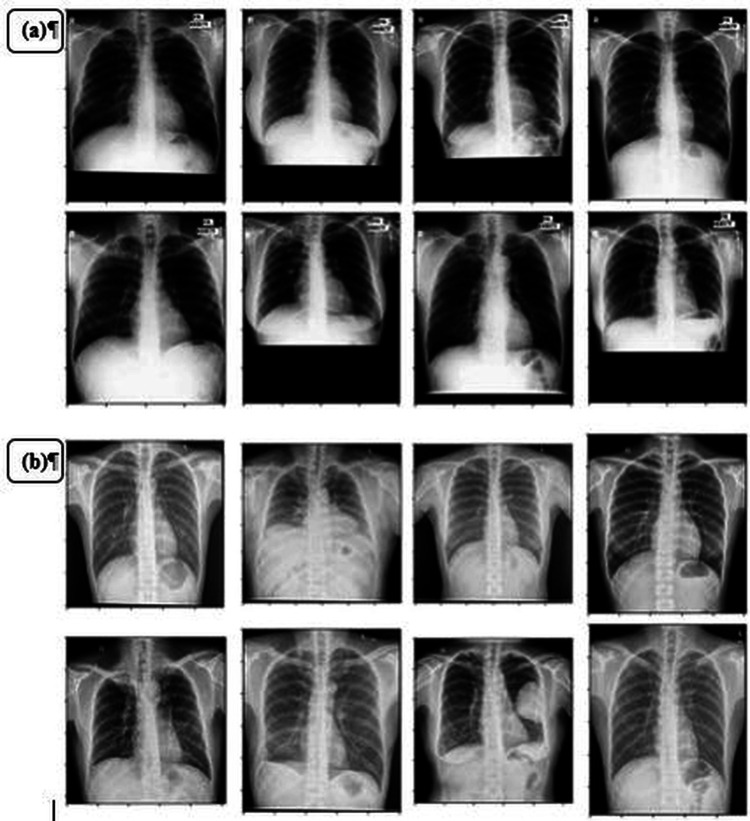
(A) Chest X-ray image samples with labels from the Shenzhen, China dataset and (B) the Montgomery County, Maryland dataset. Reproduce under the terms of the Creative Commons Attribution License from Reference [41]. Copyright 2022 Polish Medical Society of Radiology.
